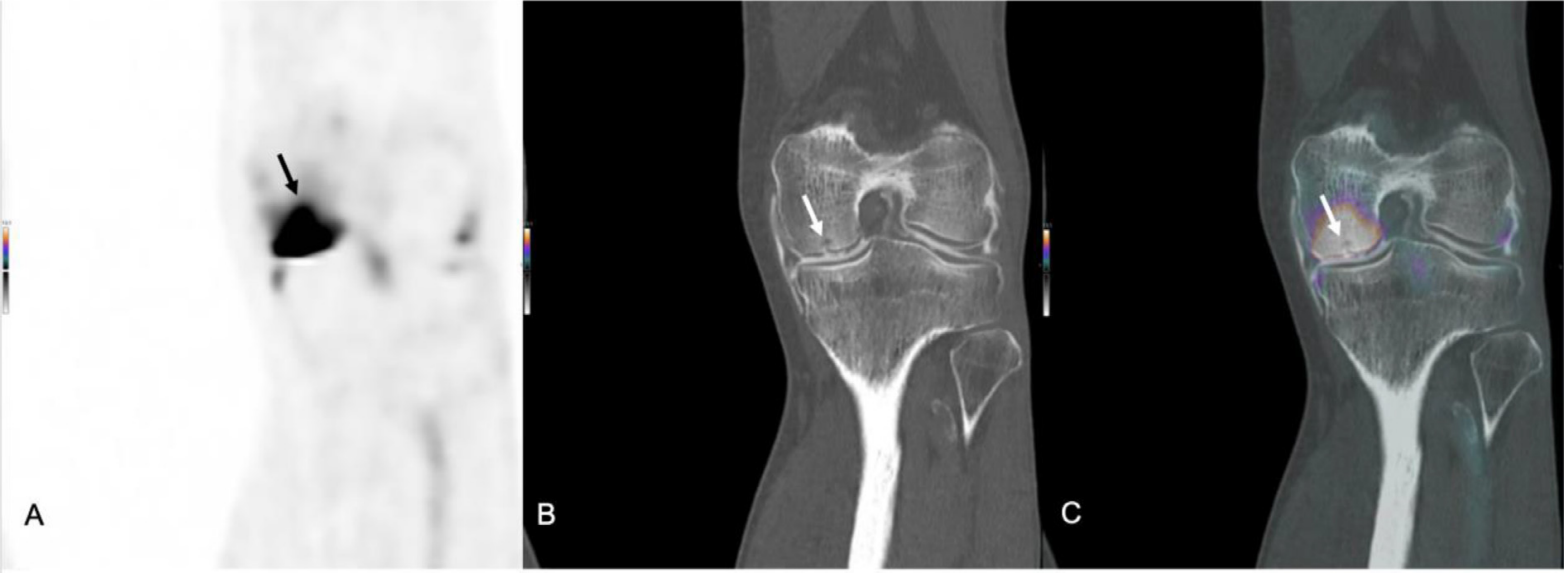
Coronal images of a sodium fluoride-18 PET/CT (Na[^18^F]F-PET/CT) scan with intra-articular contrast (A, PET; B, CT; C PET/CT fusion) with extensive subchondral cyst formation in the medial femoral condyle, accompanied by an associated cartilage defect (Grade 4) and heightened Na^18^F uptake (arrows). Osteophyte formation on the lateral side of the lateral femoral condyle, along with eminence spurring, was noted, all indicative of degeneration.
